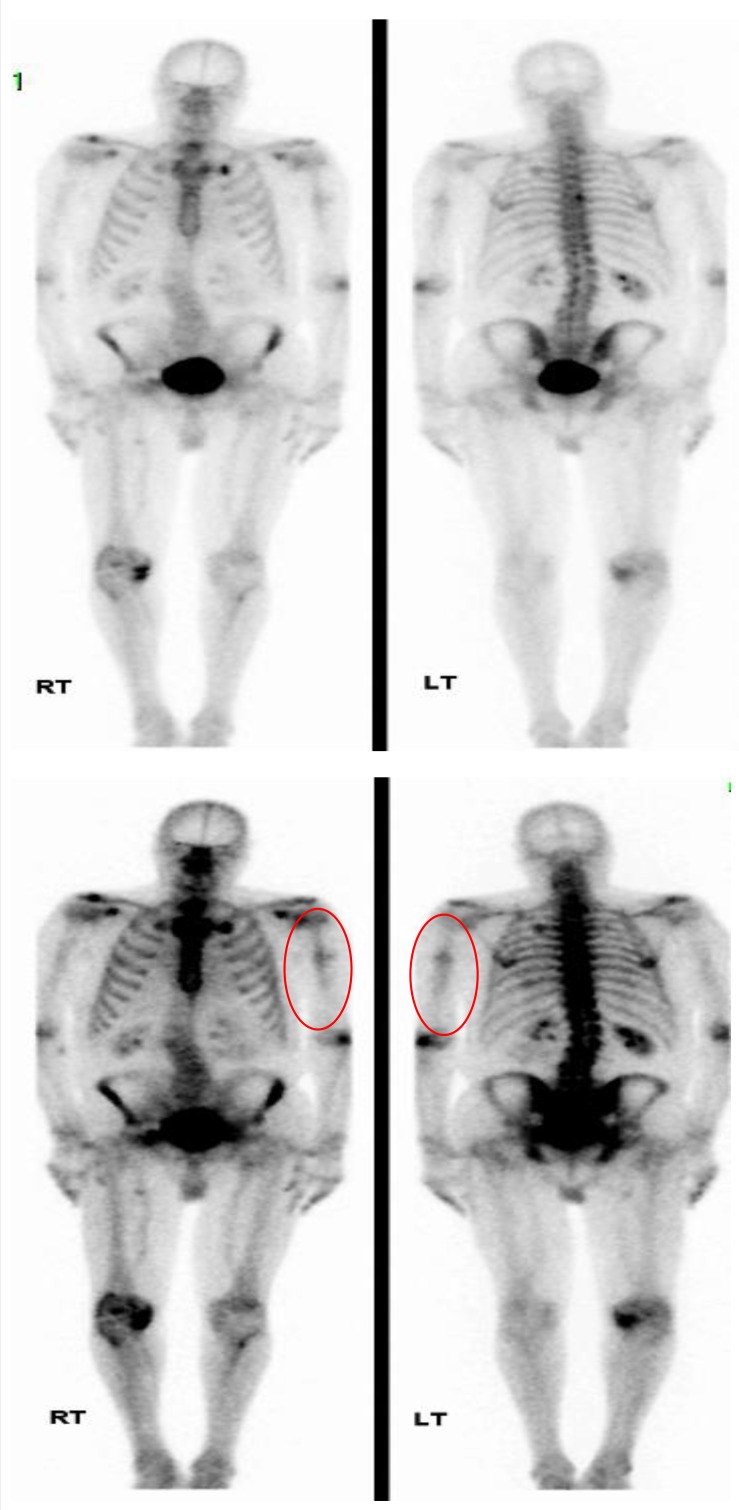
Bone scintigram revealing mild increased accumulation at the left humeral fracture site on late static bone, but no evidence of active osteomyelitis (circled in red). There is also benign degenerate uptake noted at various levels in the scoliotic spine and in the shoulder girdle, as well as the knee.
